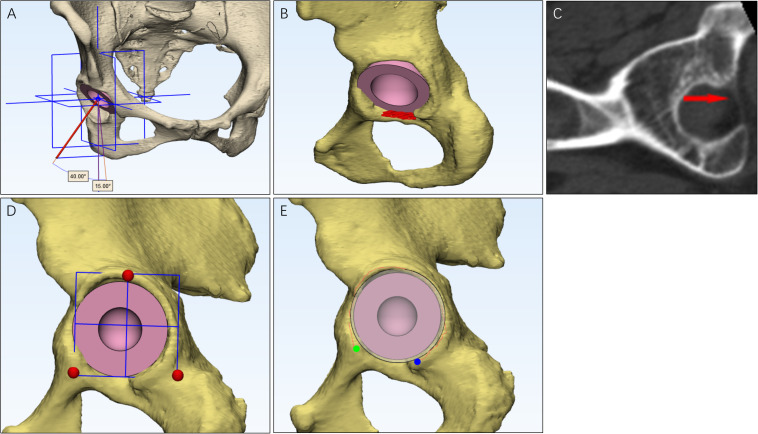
The methods to use the four cup positioning guides. For the mechanical alignment guide (A), inclination was the angle between the cup axis and the sagittal plane, and the anteversion was the angle between the longitude axis and the cup axis that was projected to the sagittal plane. For transverse acetabular ligament guide (B), the face of the cup was placed parallel to the transverse acetabular ligament, and the face was attached to the inner edge of the transverse acetabular ligament, which could be observed in CT (C). When using the ischiadic-pubis line guide (D), the face of the cup was paralleled to a plane that was consisted of three points, including the lowest point of the acetabular sulcus of the ischium, the prominence of the superior pelvic ramus, and the most superior point of the acetabular rim. When using acetabular rim labrum guide (E), the face of the cup was paralleled to the plane that is fit to the labrum around the acetabular rim.
